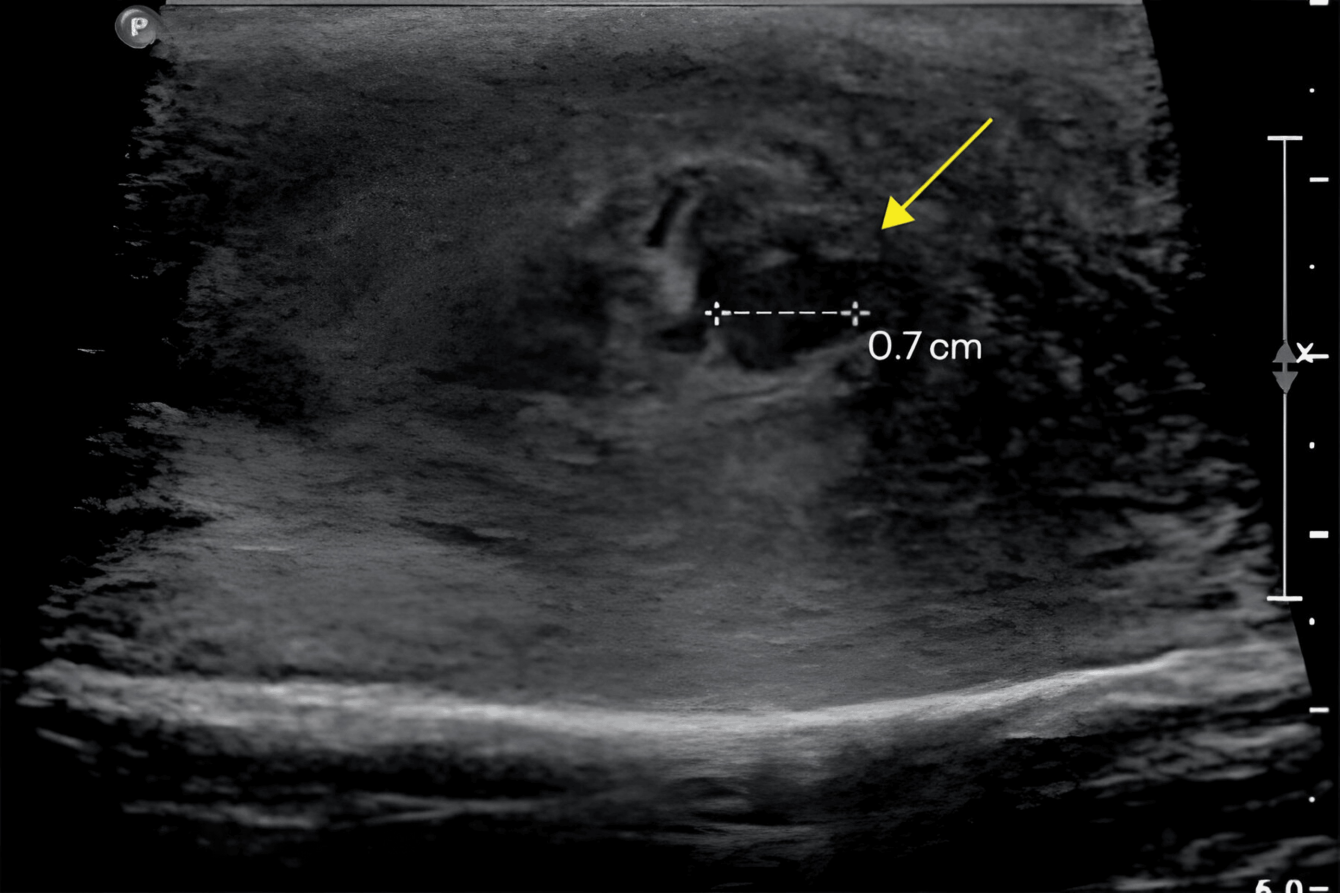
Ultrasonographic Imaging of left epididymis showing left epididymal tail collection (yellow arrow) measuring 0.7 cm with diffuse heterogeneous enlargement of the epididymal tail consistent with epididymo-orchitis.
